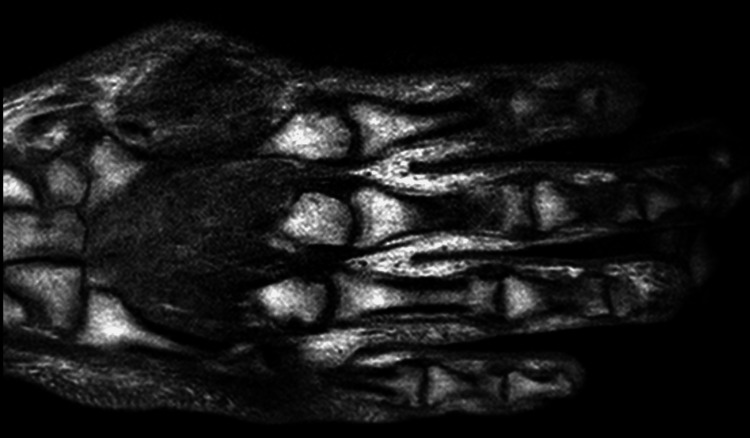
MRI left hand T1 coronal view with complex second through fourth carpometacarpal joint effusions with marked surrounding marrow edema, with moderate patchy low T1 marrow signal, suggestive of osteomyelitis and distal second phalanx osteomyelitis.
